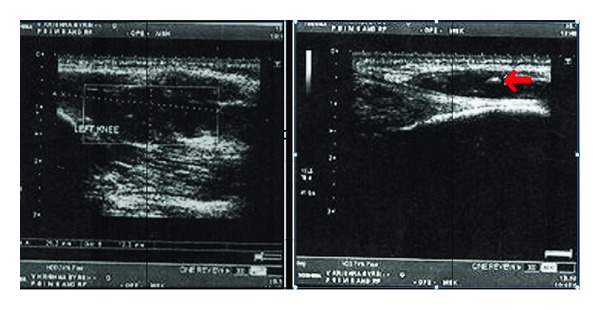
Ultrasound reveals a hypoechoic irregular mass located in the muscular plane with evidence of multiple calcific areas (marked with red arrow).
